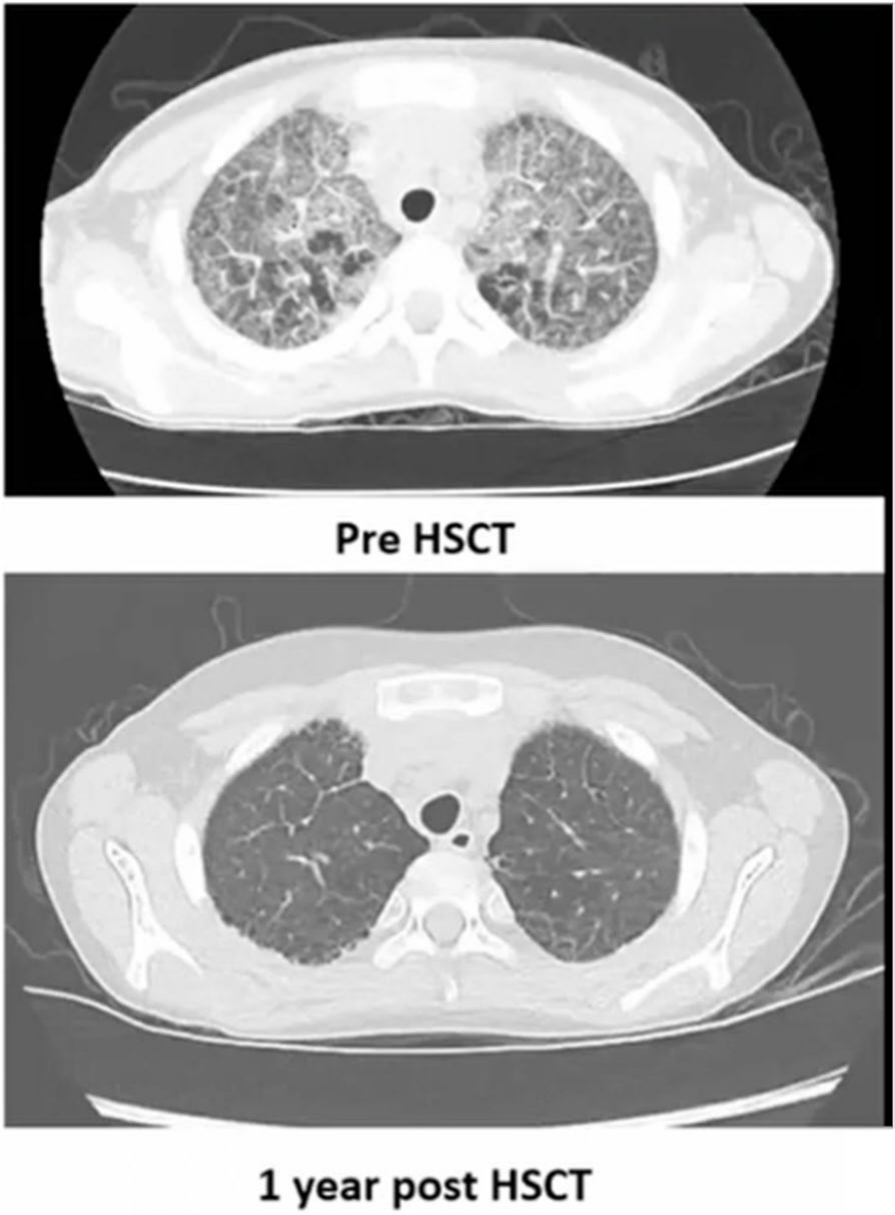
Case 6: Chest CT scan for lungs: Pre HSCT and 1-year post-HSCT
